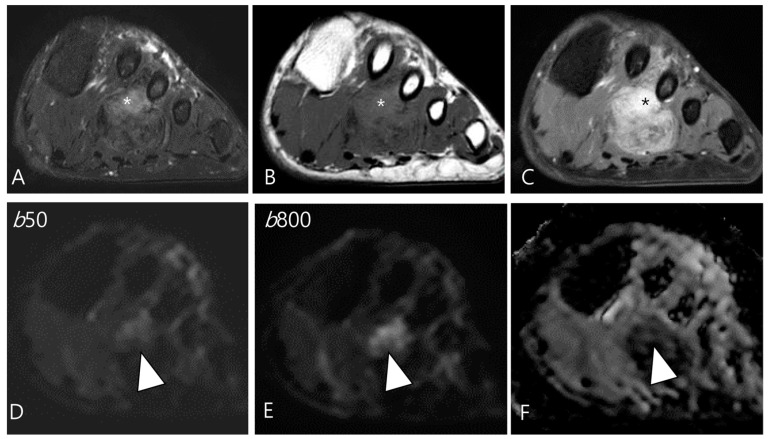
A 46-year-old male with D-TSGCT in the foot. (A) Axial fat-suppressed T2WI shows a heterogeneously high (asterisk) to low SI soft tissue mass involving the second web space. The mass extends to the plantar muscles. (B) Axial T1WI shows the mass with iso (asterisk) to low SI. (C) Axial contrast-enhanced fat-suppressed T1WI shows the heterogenous enhancement (asterisk) within the mass. (D,E) The enhancing portion of the mass shows persistent high SI on both the low and high b-value images of the DWI (arrowhead). (F) The mass shows a low ADC value (arrowhead) in this area, suggesting diffusion restriction.
